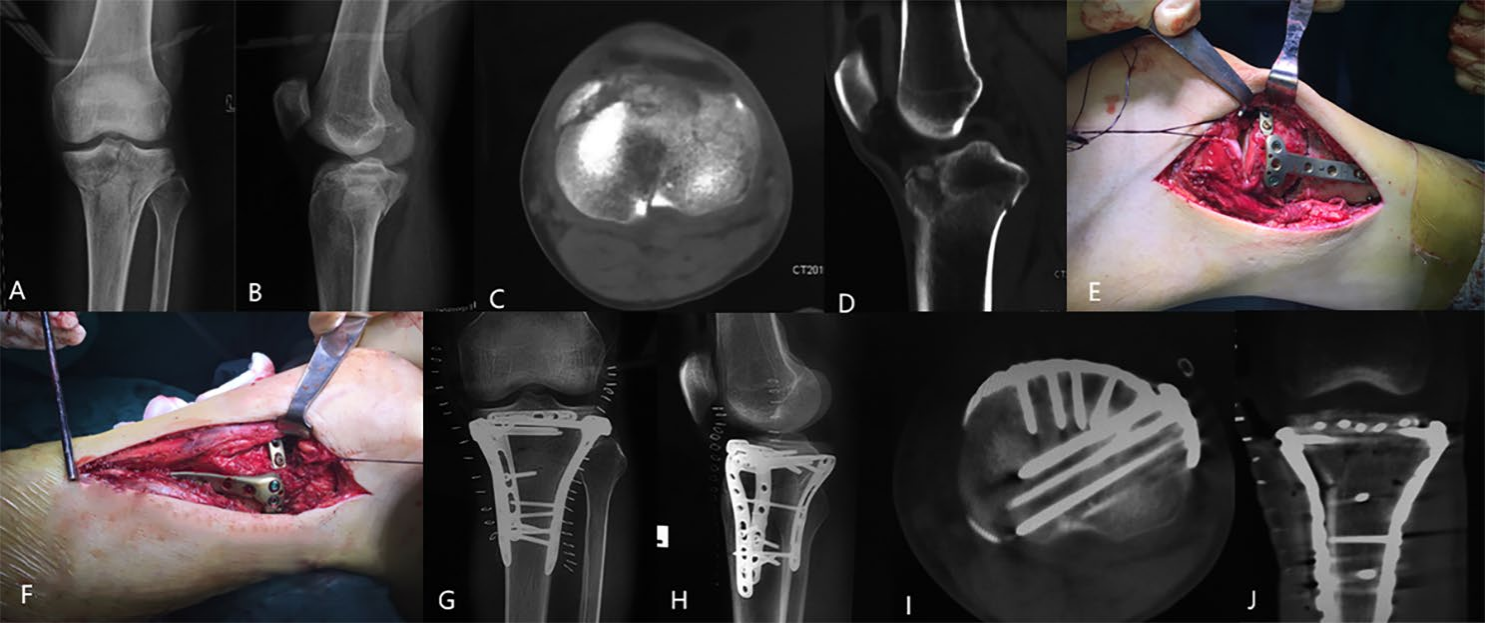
A 39-year-old male patient presented with bicondylar HTPF (A-D). To address this, a combination of the rim plate and proximal tibial locking plates was employed (E-H). The rim plate was strategically inserted behind the patella tendon, with screws placed through lifting both sides of the patella (E, F). Subsequent postoperative CT scans revealed adequate support provided to the anterior plateau (I, J)
